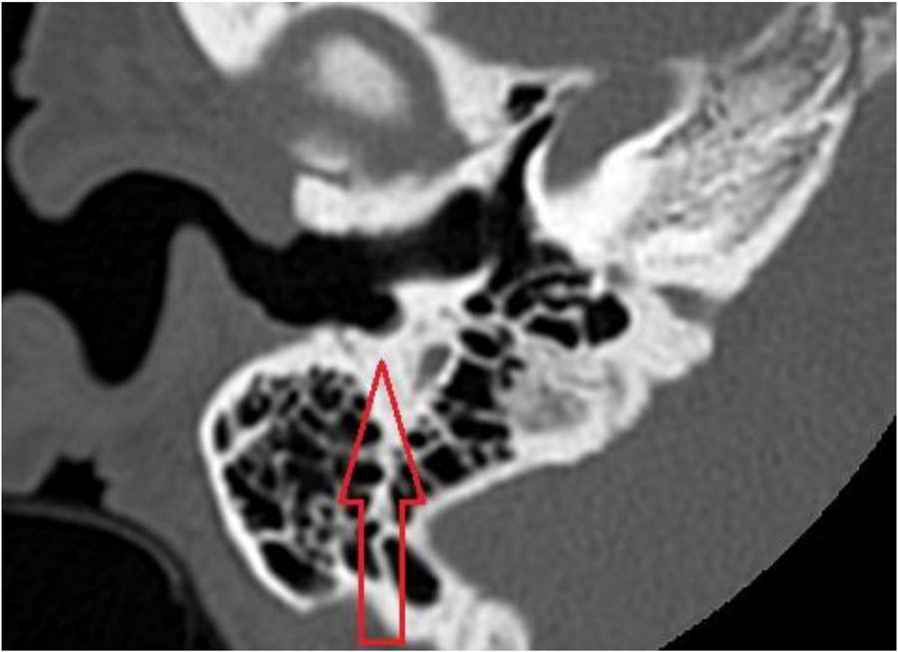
Axial cut in the inferior aspect of the external auditory canal in patient A (CT of the temporal bone) demonstrating the indentation of the inferior aspect of the external auditory canal.
